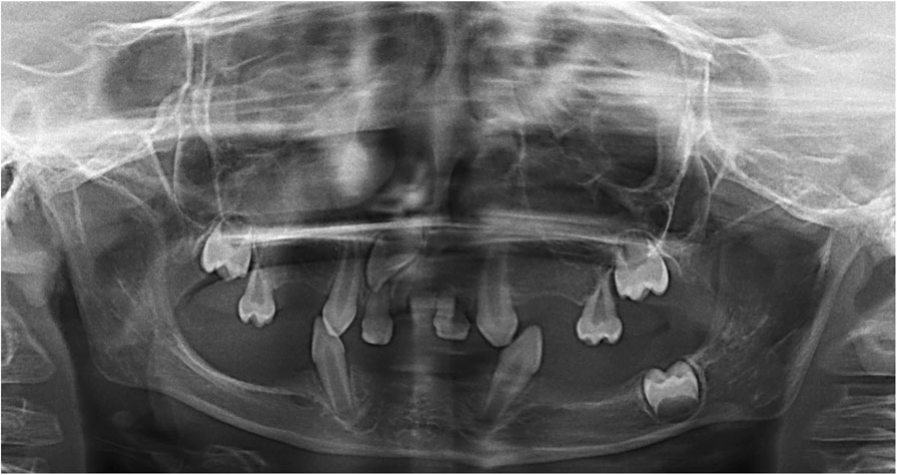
Panoramic radiograph showing the presence of four unerupted permanent tooth germs
